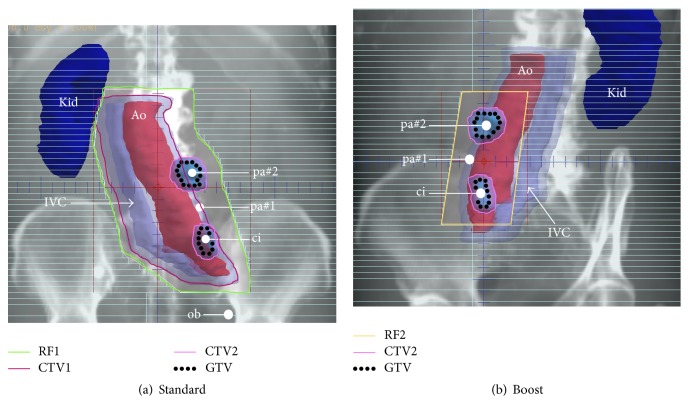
Irradiation conditions for the lymph nodes*. (a) Frontal view of CT image reconstruction; RF1 was set based on CTV1 and 2 as standard radiation. (b) Left anterior oblique view of CT image reconstruction; RF2 was set based on CTV2 as a radiation boost. CT: computed tomography, RF: radiation field, GTV: gross tumor volume, CTV: clinical target volume, Ao: aorta, IVC: inferior vena cava, Kid: right kidney, ob: obturator lymph node, ci: left common iliac lymph node, and pa: para-aortic lymph node.
